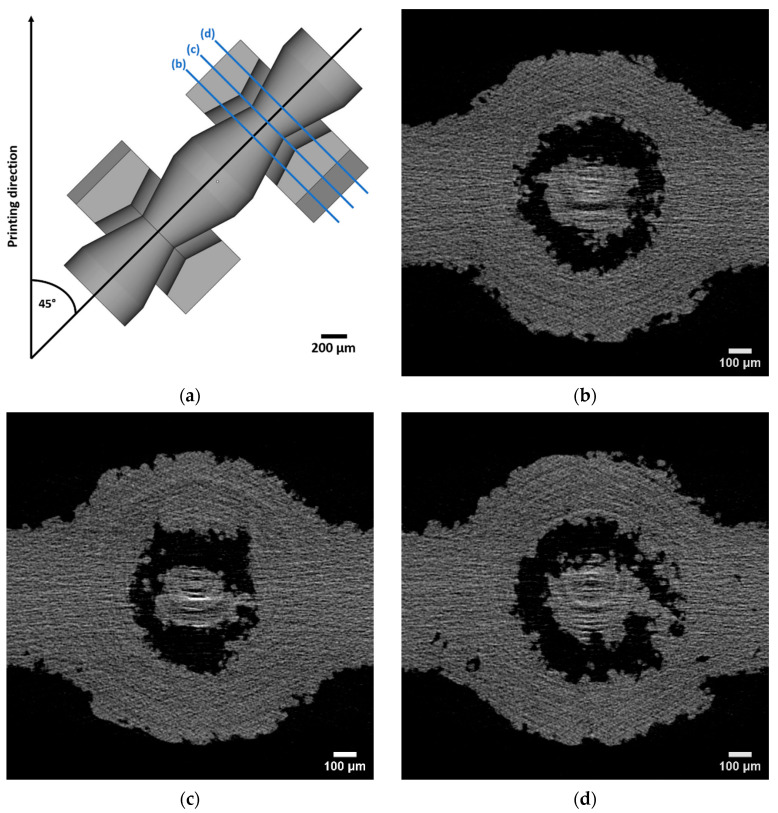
CT images of a pin-joint with a pixel size of 1.5 µm conducted on a SkyScan 1272: (a) shows the selected sections (b–d) for the CT imaging parallel to the beam plane with an averaged distance of 150 µm between the shell of the beam and these sections. The left side of the CT images point towards the building direction. The joint clearance measured by the difference of the radius using circular fit of the pin and the beam hole is (b) 115 µm, (c) 127 µm and (d) 132 µm.
